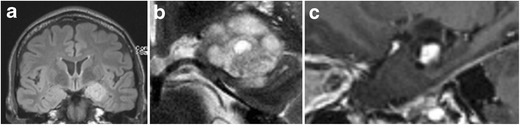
Ganglioglioma. Coronal FLAIR (a), zoomed-in coronal T2 (b) and zoomed-in sagittal contrast-enhanced 3D-MPRAGE (c) show a lobulated T2-FLAIR hyperintense space-occupying lesion in the left amygdala with focal infiltration of the left hippocampal head. The lesion contains a small central cystic (b) as well as a nodular contrast-enhancing component (c)
